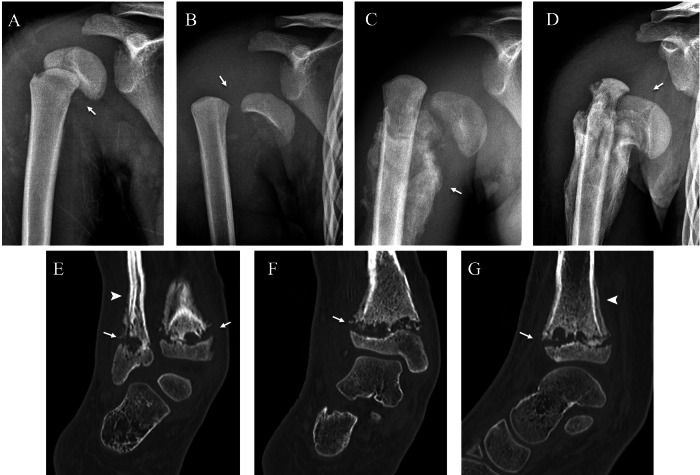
Two cases of epiphyseal separation in the right proximal humerus of a 5-year-old boy with hereditary sensory and autonomic neuropathy type IV (A–D) and the right distal tibia and fibula of a 9-year-old girl undergoing treatment for myelomeningocele (E–G). Anteroposterior right shoulder radiographs at injury (A), 1 week (B), 1 month (C), and 2 months (D) demonstrate, respectively, epiphyseal displacement (A), dislocation (B), excessive callus formation (C) and healing with resulting arm shortening (D) (arrows). Coronal (E, F) and sagittal (G) CT of the right ankle shows widening of the growth plate, irregularity and lytic areas of the metaphysis and epiphysis of the tibia and fibula (arrow), and periosteal reaction (arrowhead). Courtesy of Dr. Maher Yatim, Auxerre
